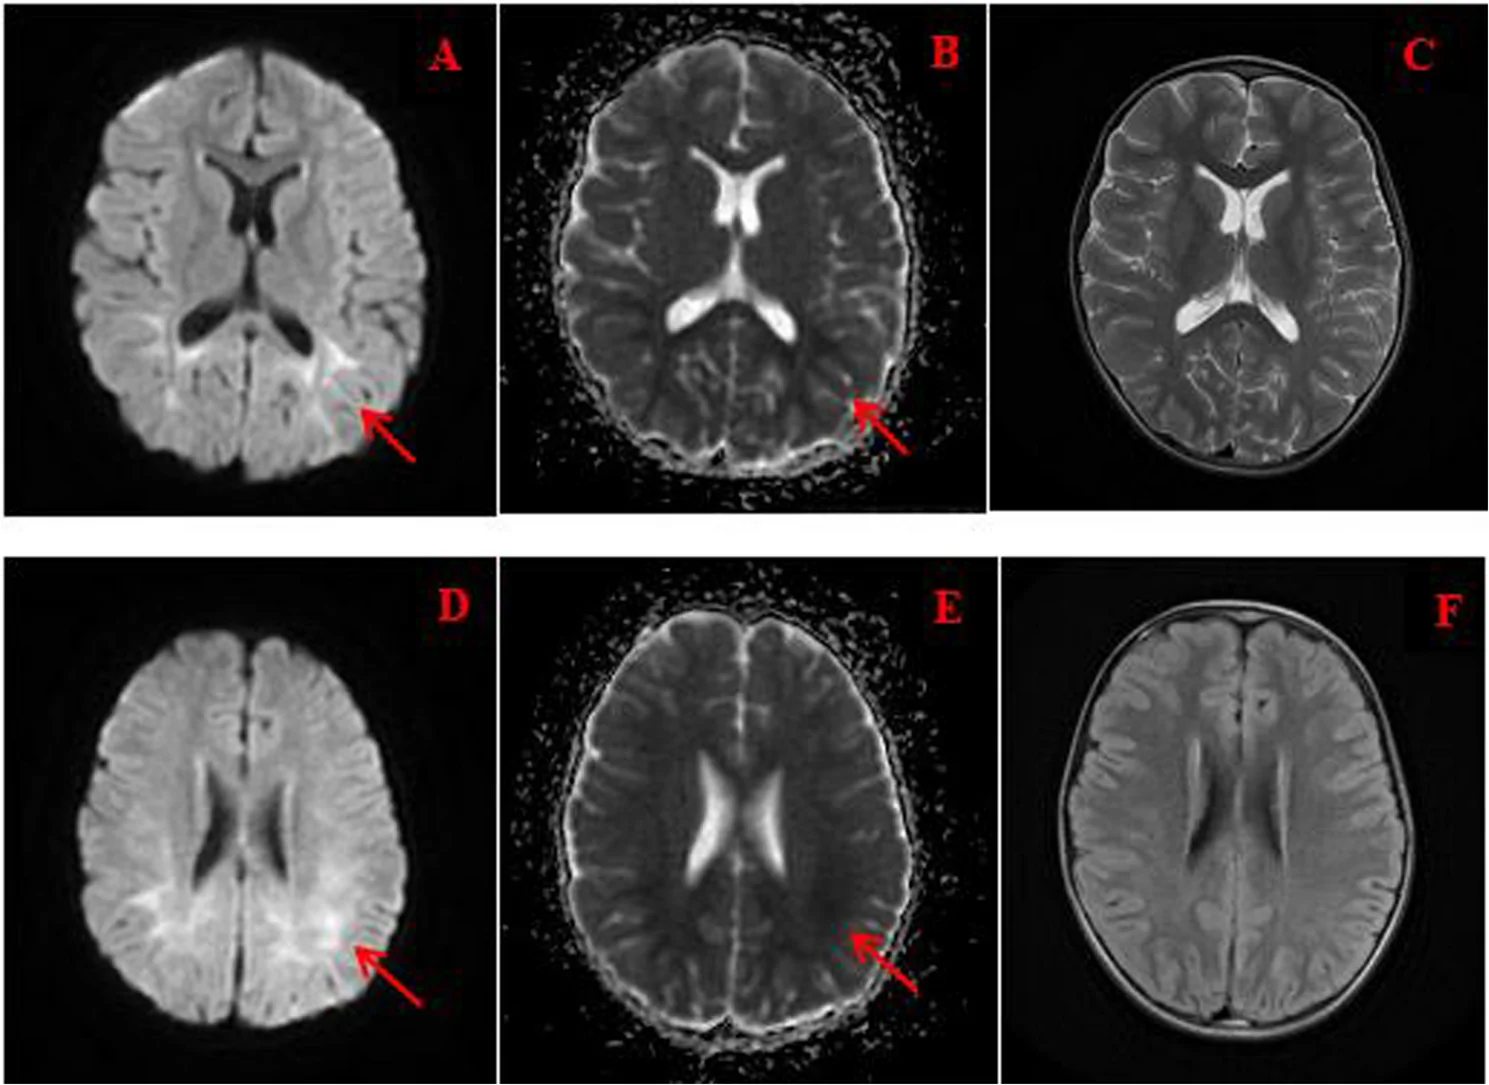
Brain MRI results of the child with hypersarcosinemia (January 12, 2025). A and D Patchy DWI hyperintense signal in the subcortical white matter of the occipital lobe of the bilateral cerebral hemispheres (arrows). B and E Patchy hypointense signal on ADC maps in the subcortical white matter of the occipital lobe of the bilateral cerebral hemispheres (arrows). C The T2-weighted image showed no abnormalities. F The FLAIR image showed no abnormalities. MRI, Magnetic resonance imaging. DWI, Diffusion-weighted imaging. ADC, Apparent diffusion coefficient. FLAIR, Fluid-attenuated inversion recovery
